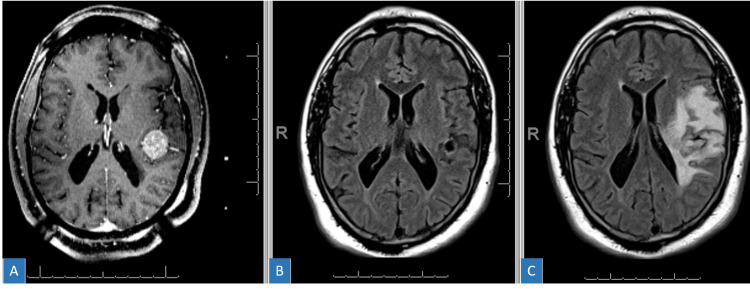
Radiation necrosis after 27 Gy in three fractions. (A) Pre-treatment planning T1 with contrast MRI. (B) Three-month post-treatment T2 FLAIR MRI with excellent response and minimal surrounding edema. (C) Six-month post-treatment T2 FLAIR MRI with clinically symptomatic edema. FLAIR = fluid attenuated inversion recovery; MRI = magnetic resonance imaging
